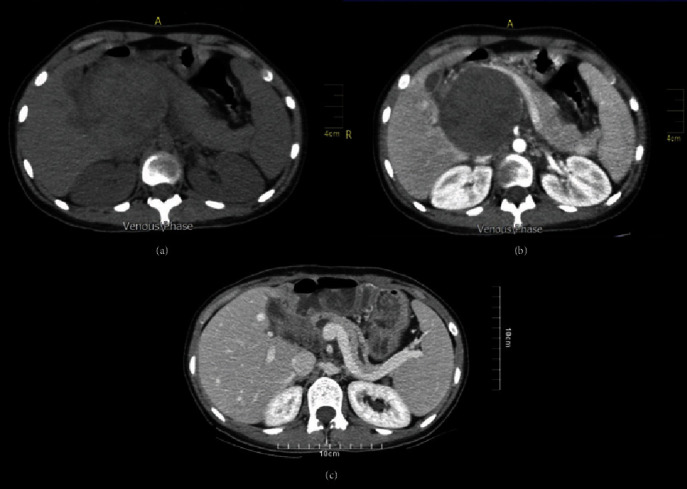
Computed tomography scans (a) without contrast and (b) with contrast before the surgery showed a hypodense mass in the epigastric area between the liver, kidney, and pancreas. (c) Postsurgical contrast-enhanced computed tomography.
